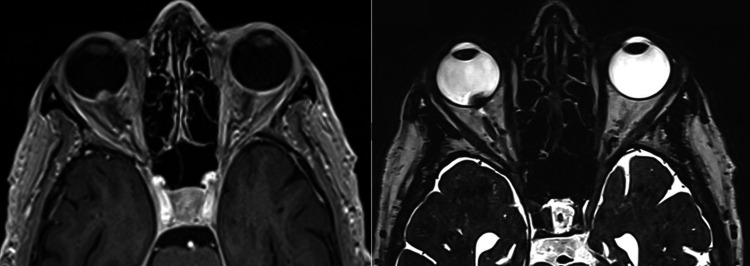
Magnetic Resonance Images - T1 (left), T2 (right). Twelve months after treatment, magnetic resonance images show a further decrease in the size of the uveal melanoma in the right eye from the initial GTV of 1.47 cm^3^ to a GTV of 0.24 cm^3^, which is a reduction of 84%. GTV: gross tumor volume
